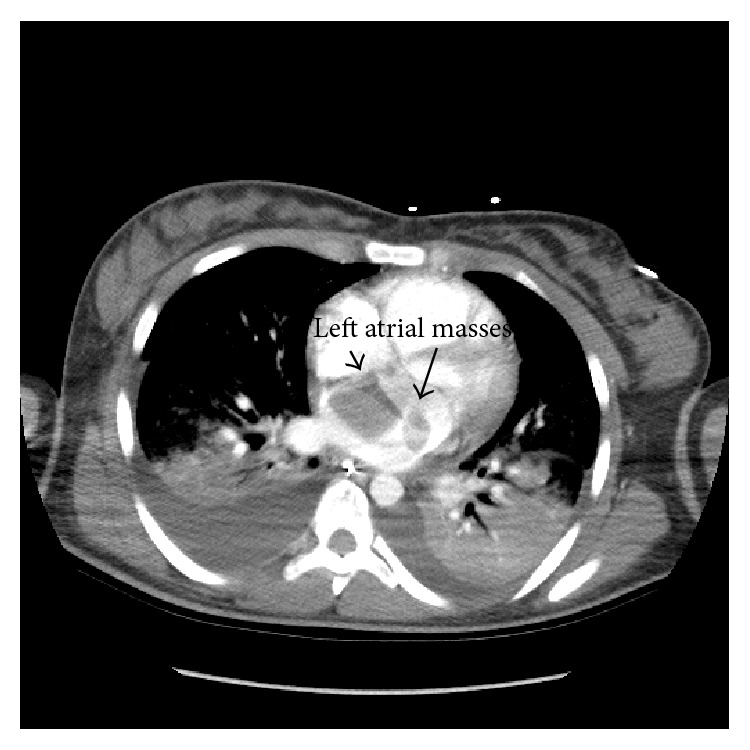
CT of the chest following intravenous contrast showing left atrial mass.
